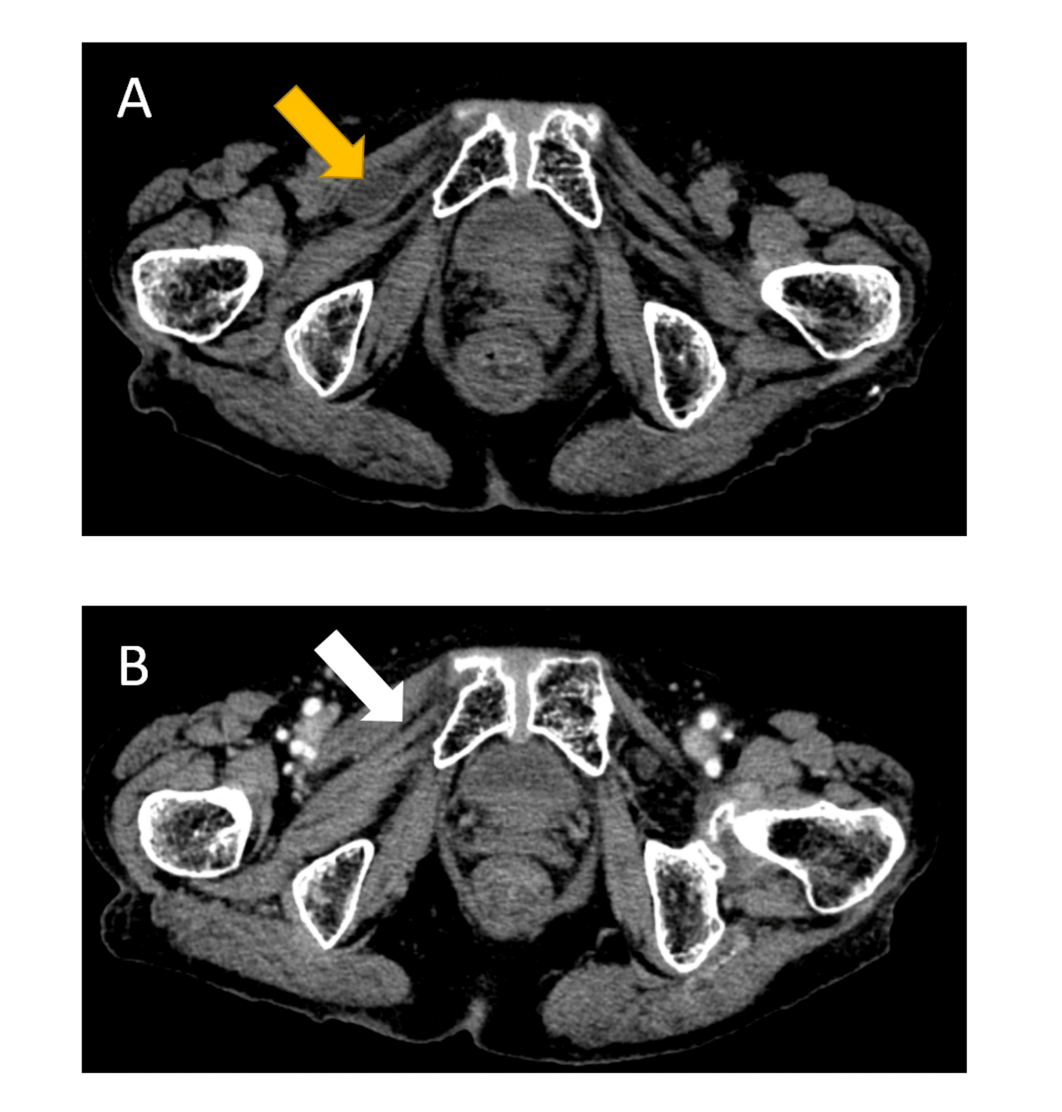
The first pelvic CT (at the onset) and the second pelvic CT (after the release of incarceration) (A) The first plain CT scan clearly demonstrated an obturator hernia presenting as a low-density round mass (yellow arrow) between the pectineus muscle and the external obturator muscle. (B) The second CT scan showed fine alleviation of intestinal packing from the obturator canal. Disappearance of a round mass (white arrow) between the two muscles was demonstrated. No intestinal ischemia was detected using a contrast medium.
